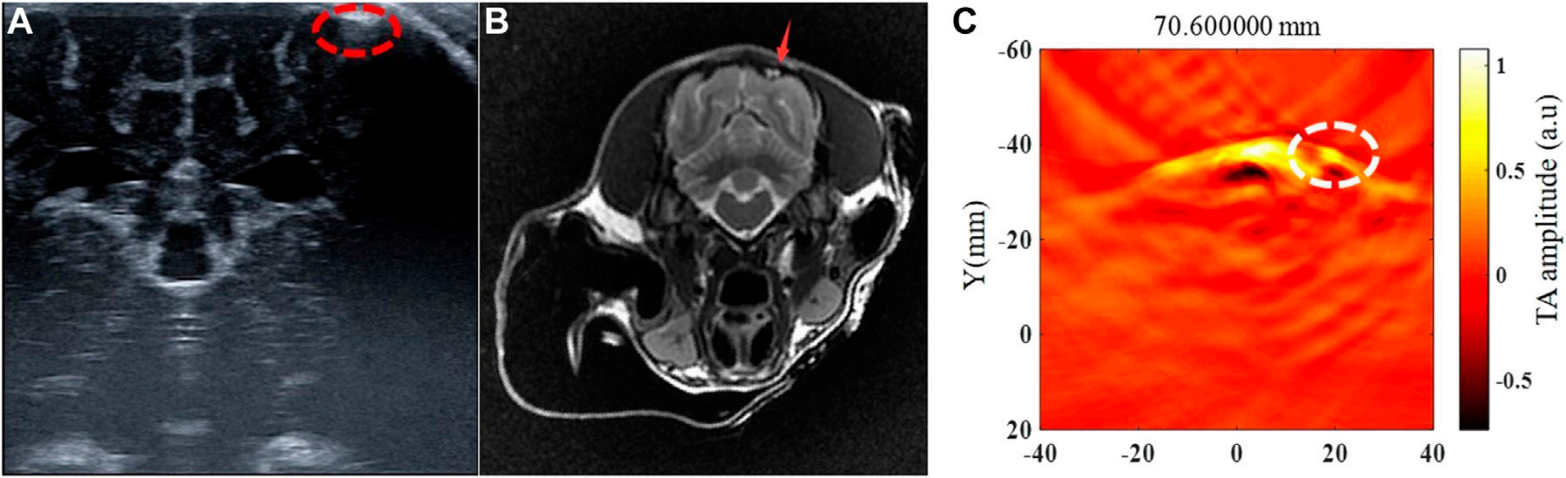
Ultrasonography, MRI and thermoacoustic imaging after 6 h of the canine intracerebral hemorrhage modeling. This is a case of canine intracerebral hemorrhage model with blood injected into cerebral sub-cortex. (A) Ultrasound showed the hemorrhage area as hyperechoic located at the left cerebral sub-cortex (red circle). (B) T2WI of MRI presented hemorrhage area as hyper-intensity located at the left cerebral sub-cortex (red arrow). (C) Thermoacoustic imaging showed a hyper-intensity of the hemorrhage area at the same location as presented at ultrasound and MRI (white circle).
